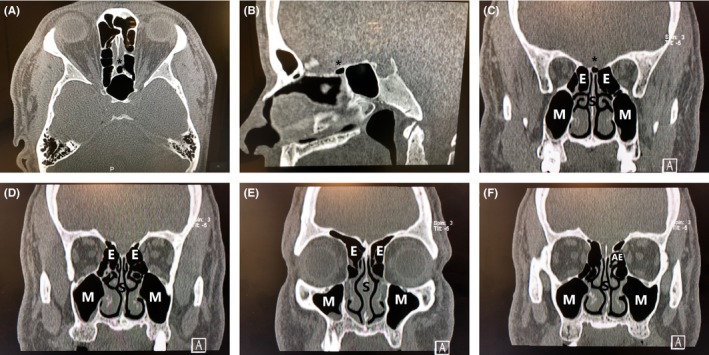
A, Axial computed tomography image of the left supraseptal ethmoid cell (labeled with star/*adjacent to the cell). B, Sagittal computed tomography image of the left supraseptal ethmoid cell (labeled with star/*superior to the cell). C, Coronal computed tomography image of the left supraseptal ethmoid cell (labeled with star/*superior to the cell). D, Coronal computed tomography image showing mucosal thickening/chronic rhinosinusitis in the right maxillary and ethmoid sinuses. E, Coronal computed tomography image showing mucosal thickening/chronic rhinosinusitis in the right greater than left maxillary and ethmoid sinuses. F, Coronal computed tomography image showing the left anterior ethmoid artery (AE) in the left ethmoid sinuses. M, maxillary sinus; E, ethmoid sinus; S, septum
